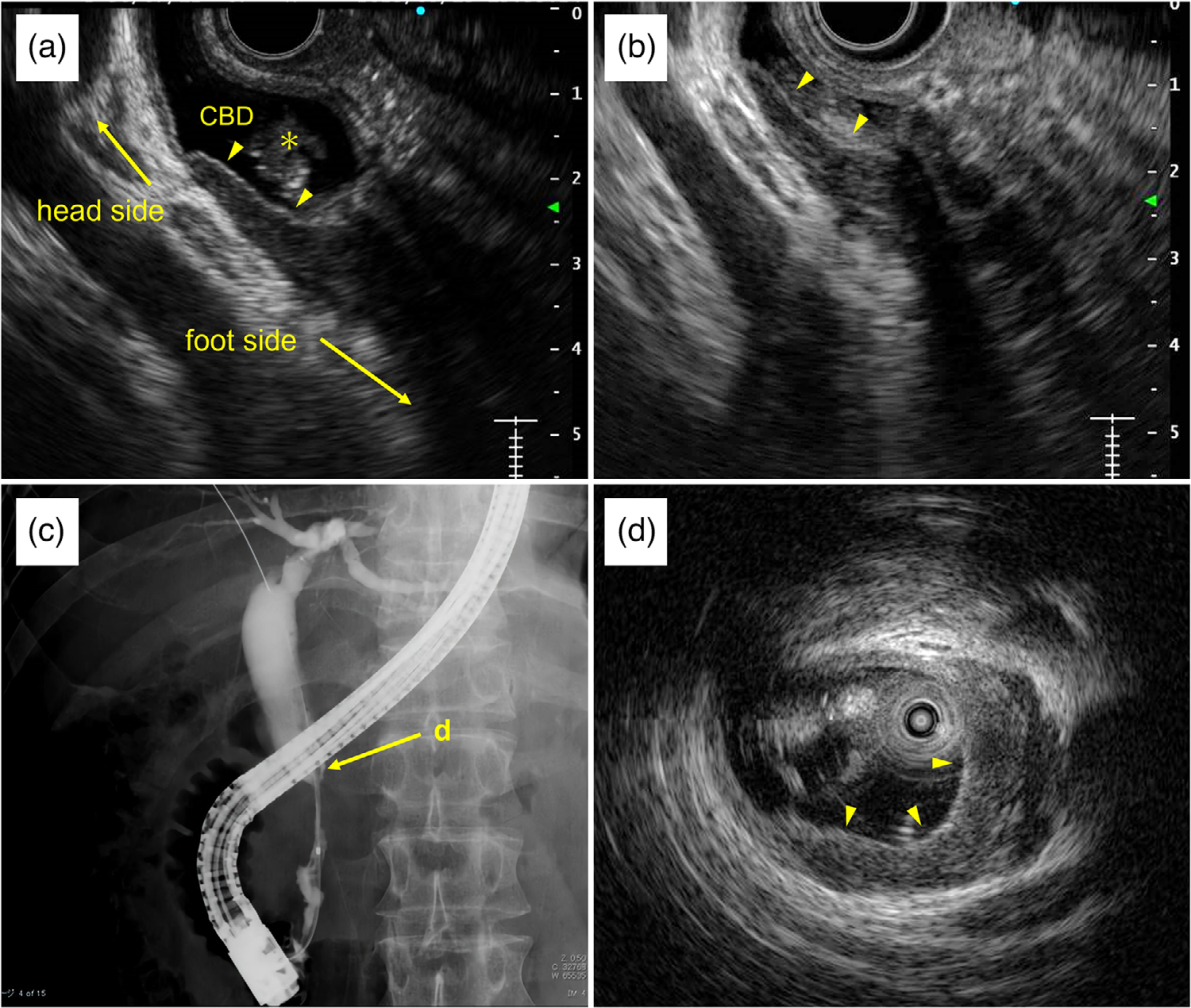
Findings of endoscopic ultrasonography (EUS), endoscopic retrograde cholangiography (ERC), and intraductal ultrasonography (IDUS): (a, b) EUS reveals irregular thickening of the inner hypoechoic layer without the disappearance of the innermost thin hyperechoic layer (arrow). A hyperechoic mass indicating a bile duct stone is also detected (*). (c) ERC shows the distal bile duct stricture with a dilated proximal bile duct. (d) IDUS of the bile duct stricture site shows irregular thickening of the inner hypoechoic layer without the disappearance of the innermost thin hyperechoic layer, as seen on endoscopic ultrasonography.
